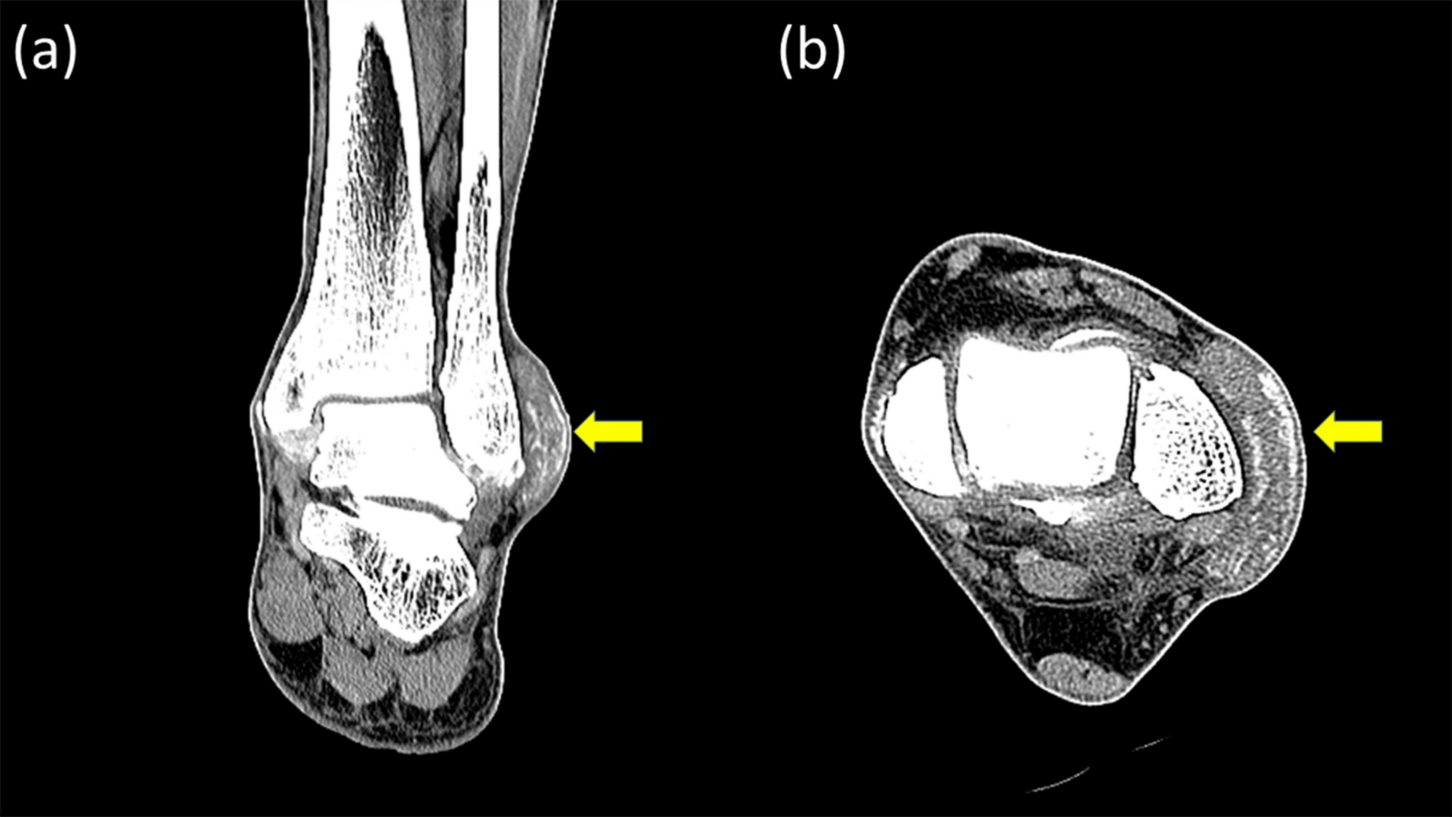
Preoperative computed tomography (a) Coronal and (b) axial computed tomography images demonstrate a nodular extra-articular soft tissue mass located directly over the lateral malleolus (arrow). Focal calcified deposits are observed along the surrounding ligamentous structures, including both lateral and medial ligaments. No bone erosion or definite intra-articular extension is identified.
